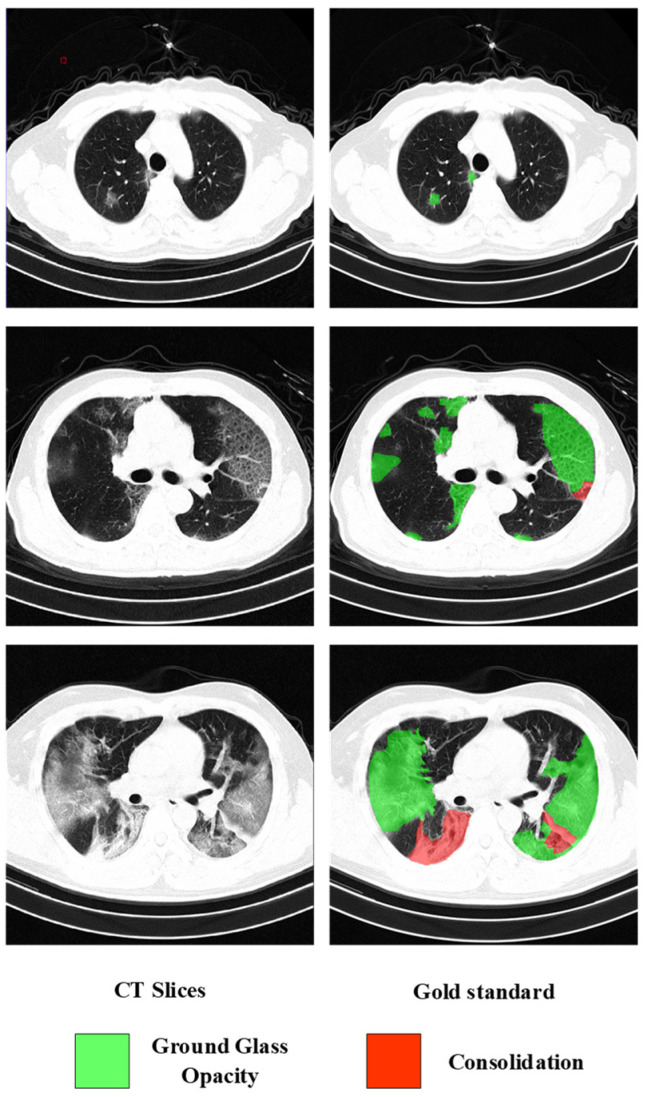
Three examples of CT images of different severity.
